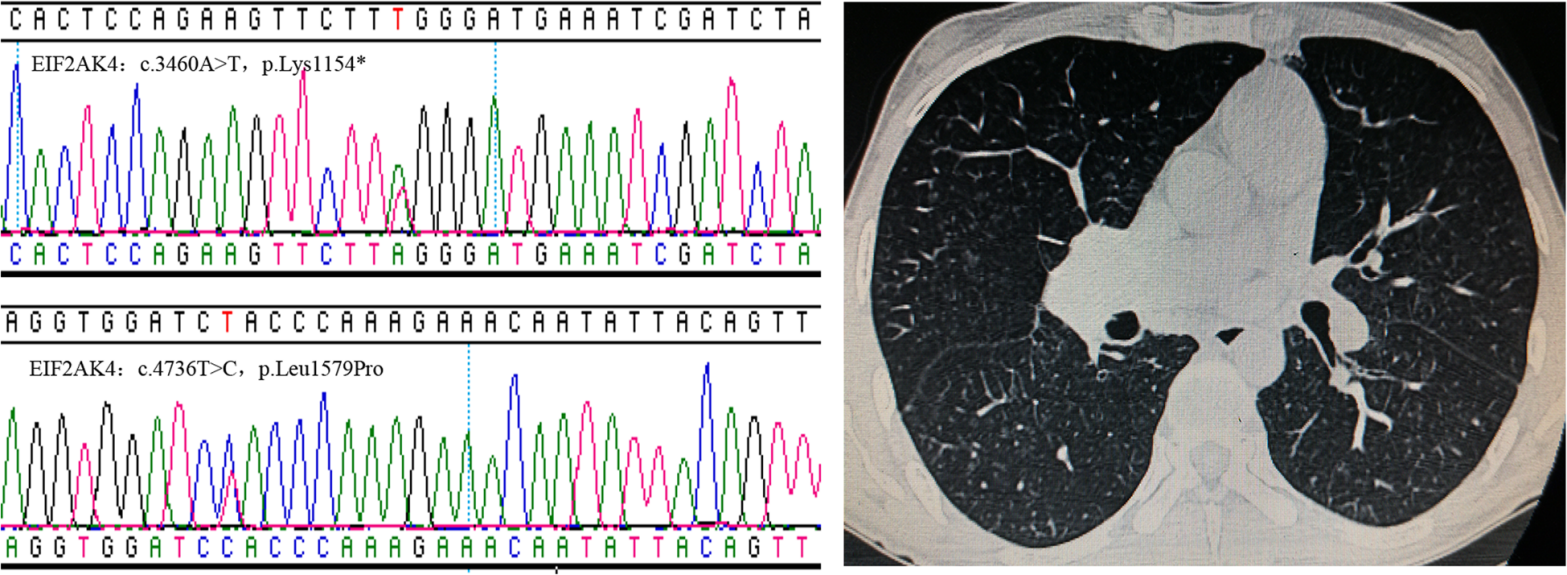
Representative images of PVOD patients. EIF2AK4 gene mutation sites (left) and chest computed tomography displaying interlobular septal thickening and ground glass opacities (right)
